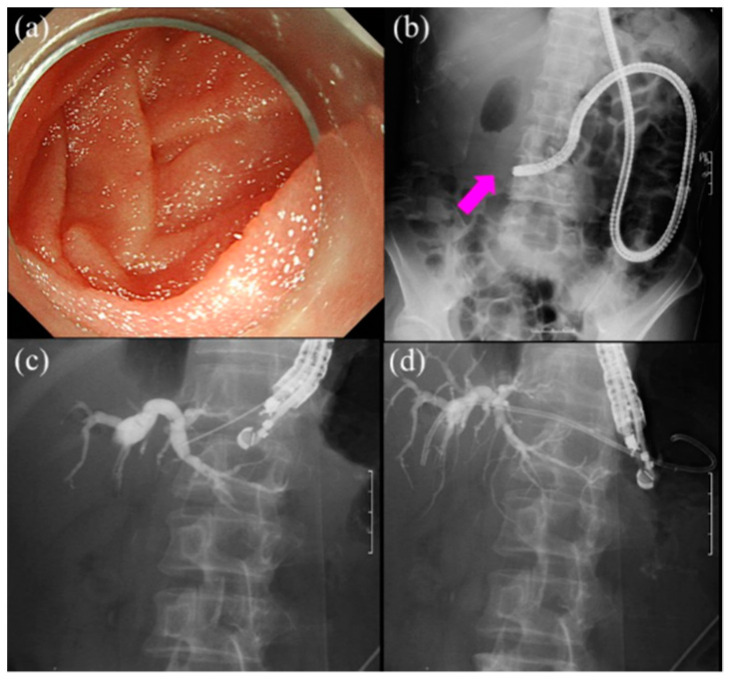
Endoscopic ultrasound-guided hepaticogastrostomy for patients with surgically altered anatomy (SAA) showing a failed case of single-balloon enteroscopy-assisted (SBE) endoscopic retrograde cholangiopancreatography (ERCP). (a) It was impossible to reach the papilla due to cancer invasion of the duodenum. (b) Fluoroscopic image showing duodenal obstruction due to cancer invasion (pink arrow). (c) Endoscopic ultrasound-guided hepaticogastrostomy is performed. First, B 3 is punctured using a 19-gauge needle. After puncture, we performed cholangiography to confirm the position of the guidewire. (d) Finally, a biliary stent was placed.
